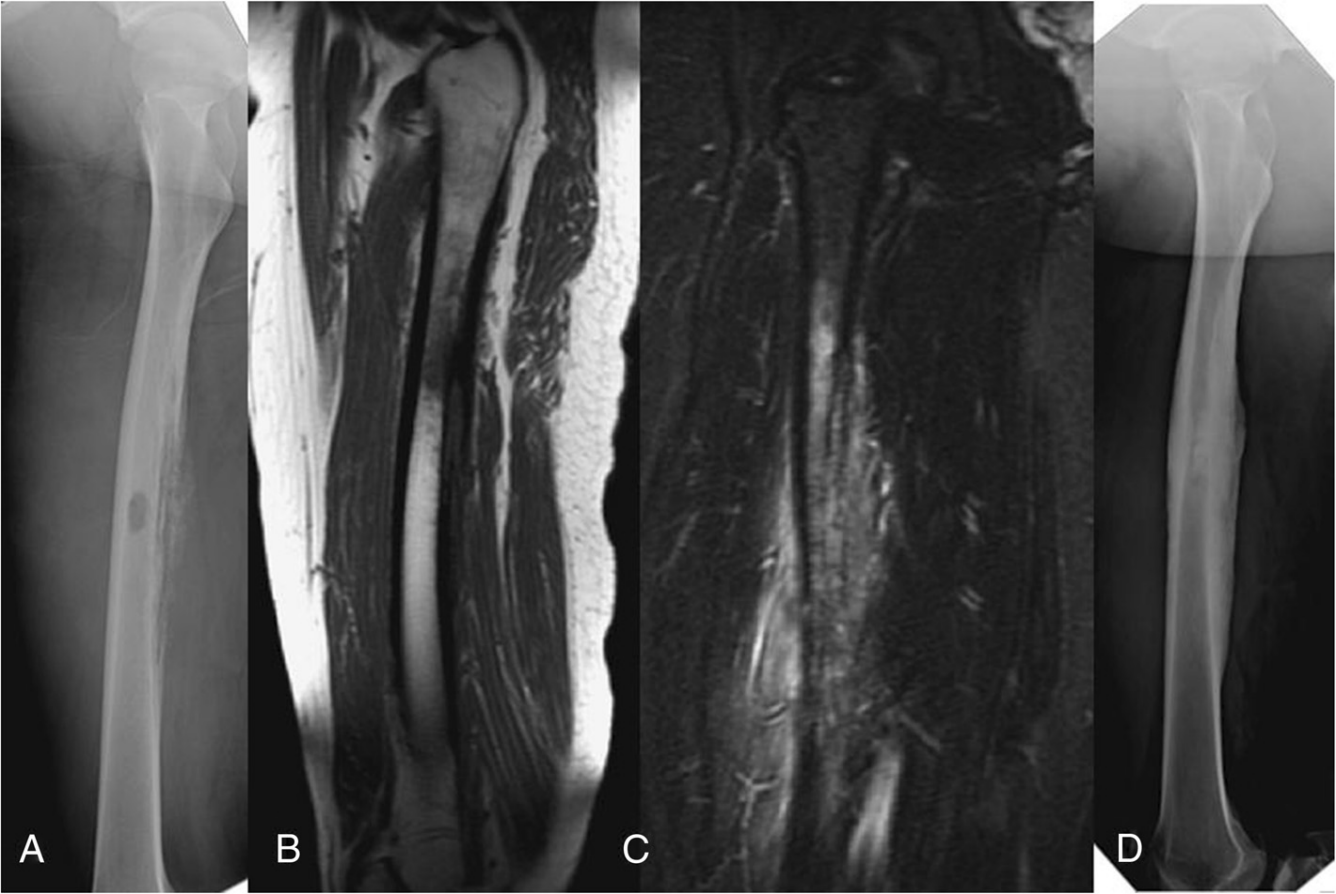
A 30-year-old healthy man who complained of moderate nocturnal thigh pain for four months after he underwent a bone biopsy. (A) A plain film of the femur showing laminated periosteal reaction with cortical destruction. (B, C) Magnetic resonance imaging (MRI) showing cortical destruction with bone marrow and soft tissue edematous change. (D) A plain film taken one year after the surgery showing bony union without recurrence.
